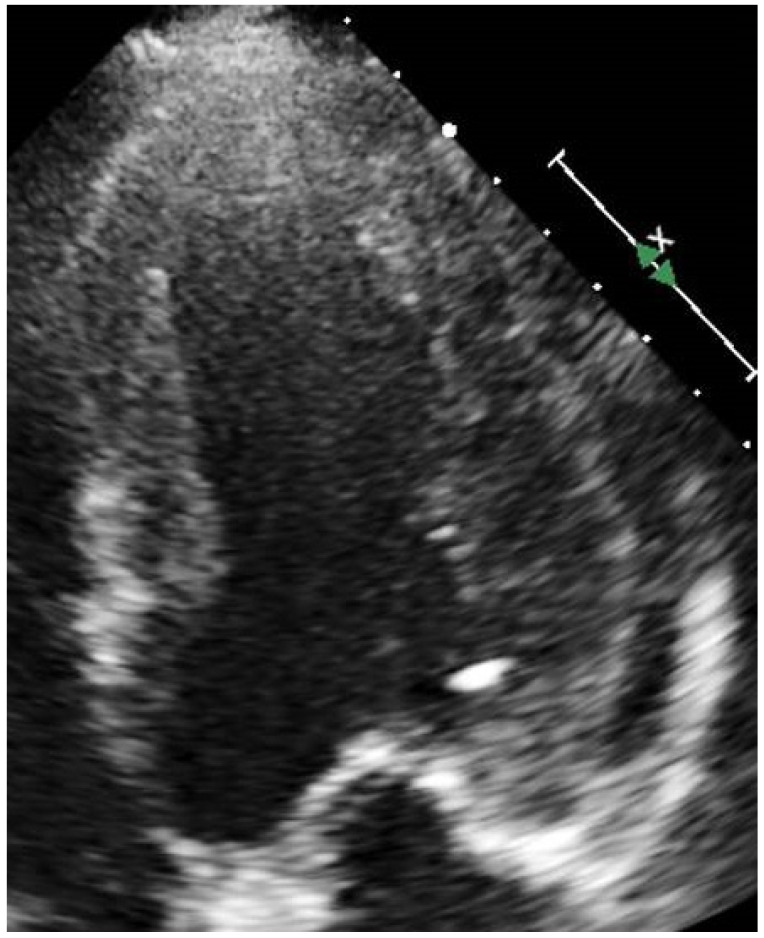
TTE apical 4-chamber view end-systole demonstrating concentric left ventricular hypertrophy (LVH).
